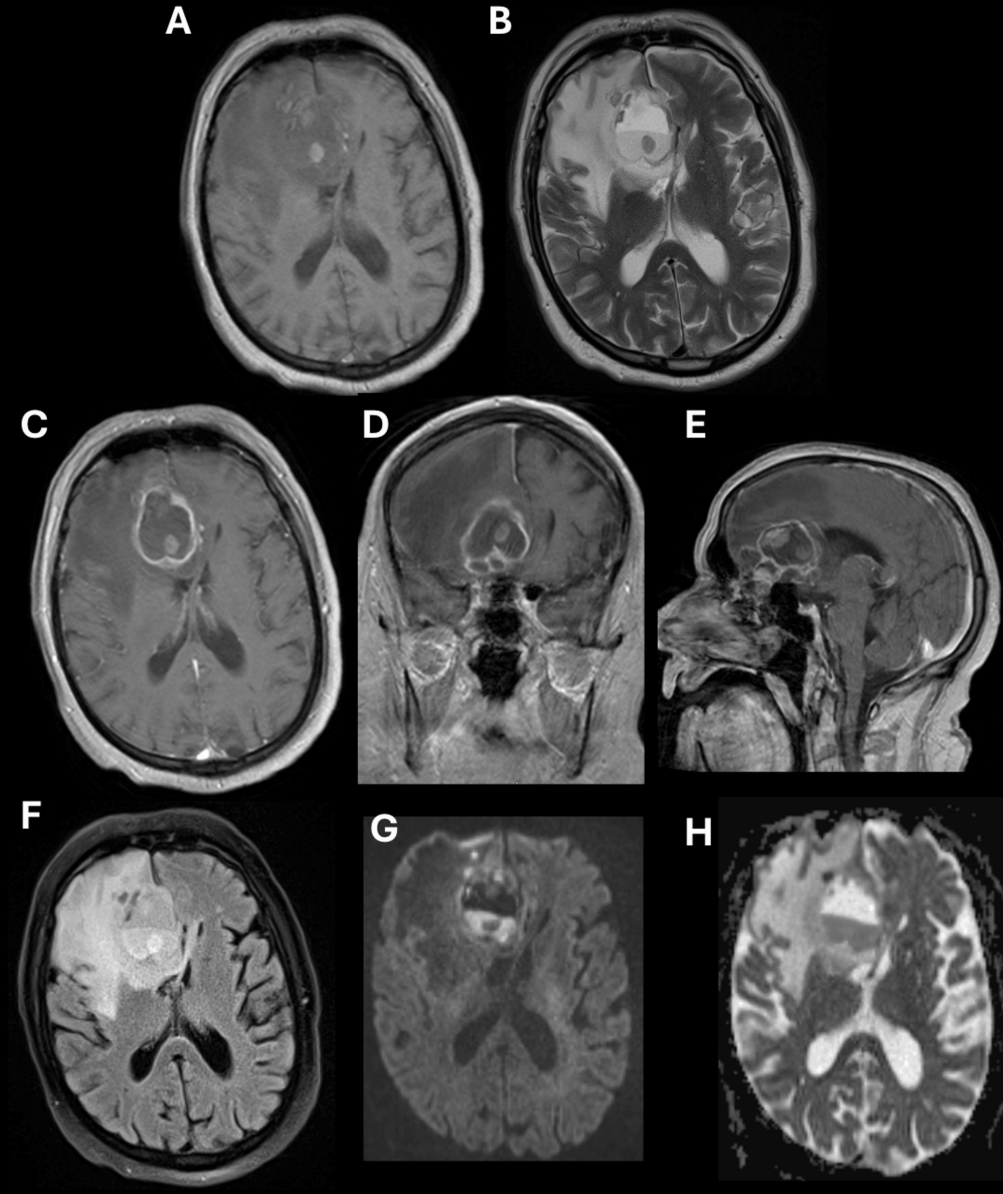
Pre-operative MRI brain MRI of the brain demonstrates a multiloculated, partially cystic, partially nodular mass with some intrinsic T1 hyperintensity (A) and heterogeneous T2 signal within the mass, with marked confluent T2 hyperintensity surrounding the mass (B). The mass demonstrates thin peripheral enhancement on T1 post-contrast images. There is also intrinsic T1 hyperintensity in the mass, suggesting fat or hemorrhagic product (C-E). For example, note the T1 hyperintensity within the mass on pre-contrast T1 images (A), which does not demonstrate enhancement on the post-T1 post-contrast images (C). Vasogenic edema in the right frontal lobe is manifest on axial FLAIR images (F). This is not secondary to infarct or a highly cellular process, as there is no associated diffusion restriction (G) or loss of ADC signal (H). Vasogenic edema is presumed secondary to inflammatory changes resulting from cyst rupture. MRI: Magnetic resonance imaging; FLAIR: Fluid-attenuated inversion recovery; ADC: Apparent diffusion coefficient; CT: Computed tomography
